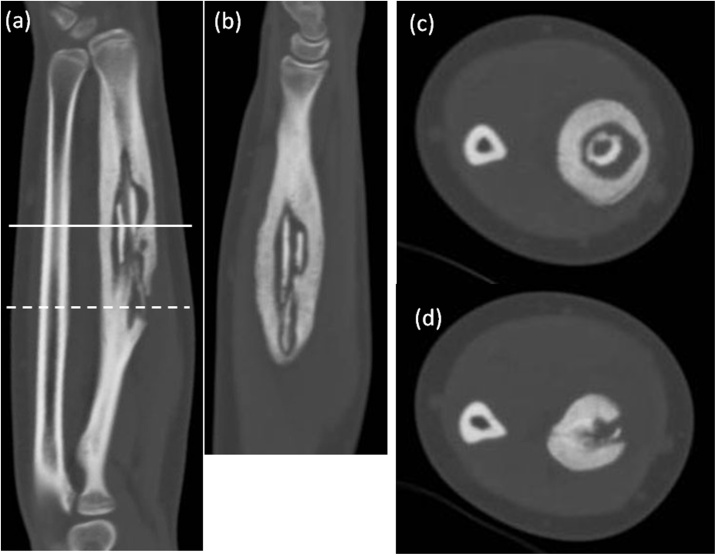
Plain CT. A CT scan shows a longitudinal translucent lesion, a cortical defect of the middle diaphysis, and cortical bone in the bone marrow of the radius. The solid and dotted lines in figure (a) correspond to figure (c) and figure (d), respectively.
